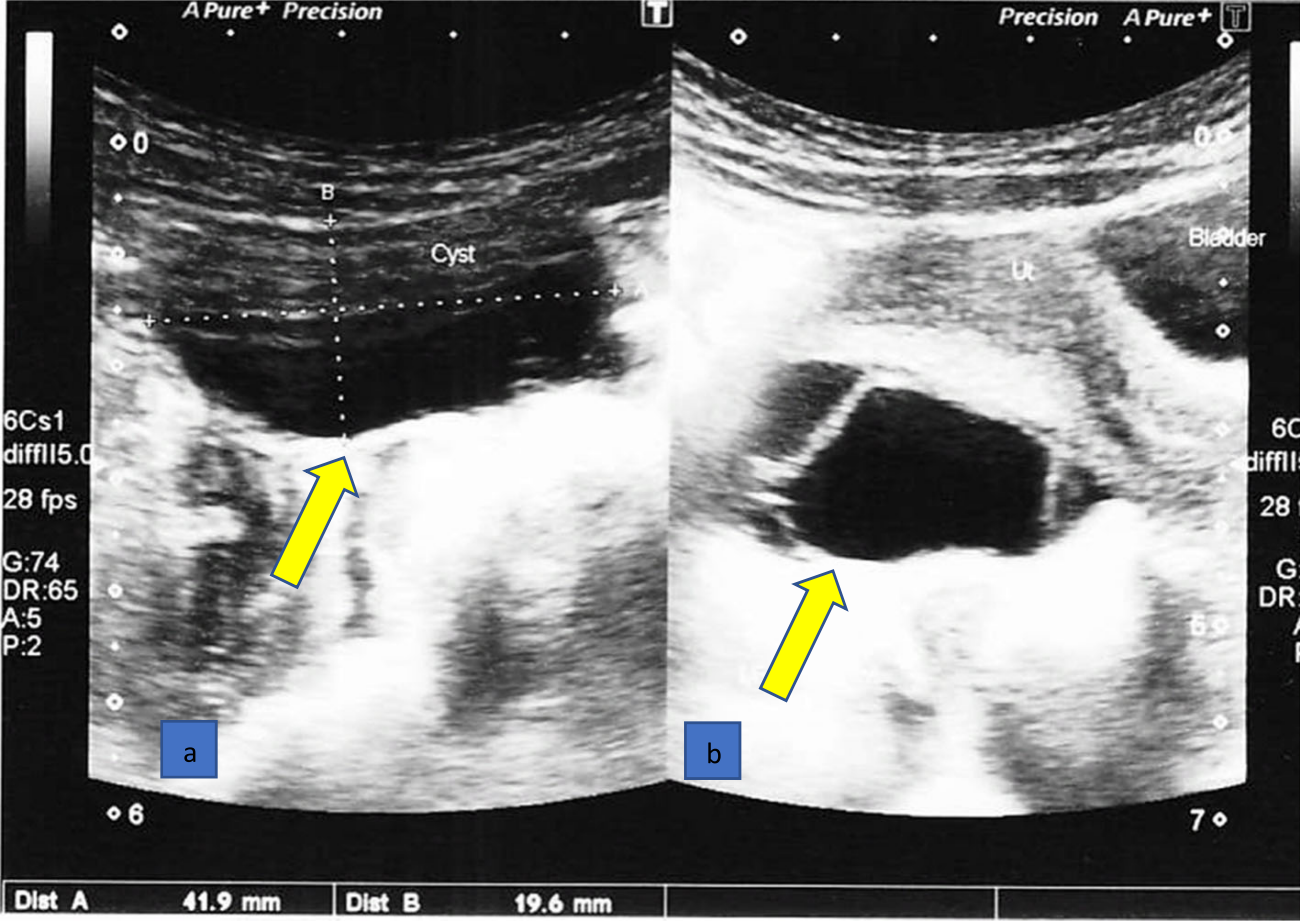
Ultrasound scan image of a 3.3 cm × 2.1 cm × 2.5 cm sized complex cyst (yellow arrow) (a) and enlarged left ovary (yellow arrow) (b)
